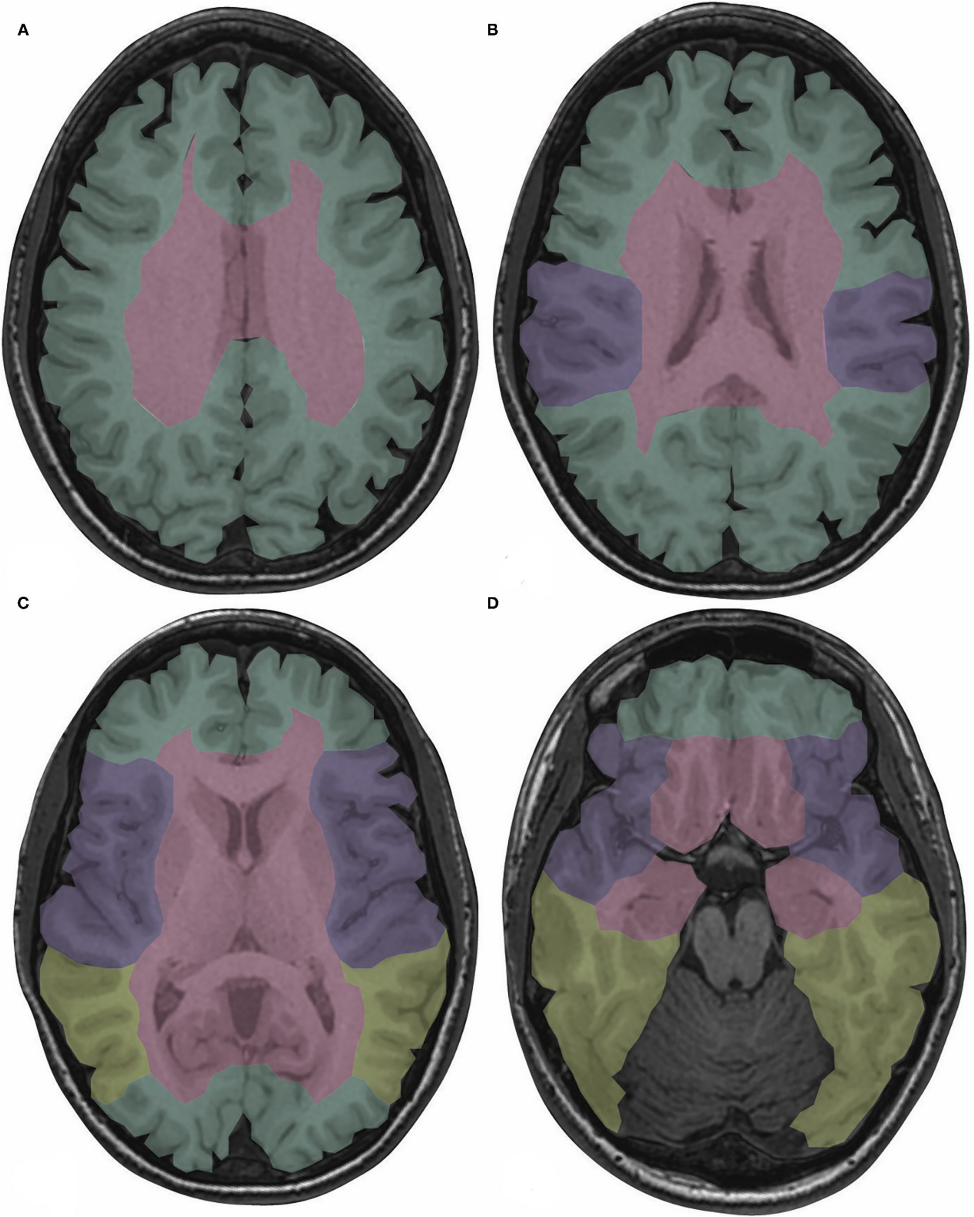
Drainage territories of the cerebral venous system are color-coded on T1-weighted images depicting normal brain anatomy at the levels of the centrum semiovale (A), body of the lateral ventricles (B), basal ganglia (C), and midbrain (D). The superficial/cortical veins drain the cortex, subcortical white matter, and SSS (green). The deep cerebral veins drain the basal ganglia, thalami, internal capsules, and third and lateral ventricles (red). The sphenoparietal and cavernous sinuses drain the peri-Sylvian region (purple). The TS and VL, if dominant, drain the posterior temporal and inferior parietal lobes (yellow) (5, 7). SSS, superior sagittal sinus; TS, transverse sinus; VL, vein of Labbé.
